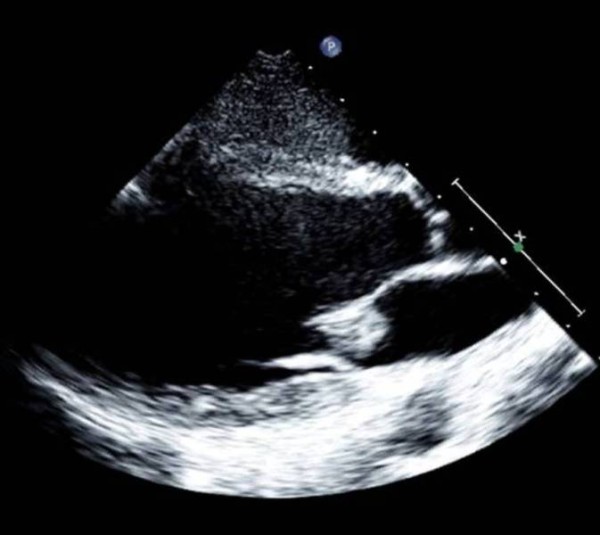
Transthoracic echocardiogram showing a mitral vegetation of 15 × 13 mm on the anterior cusp.
